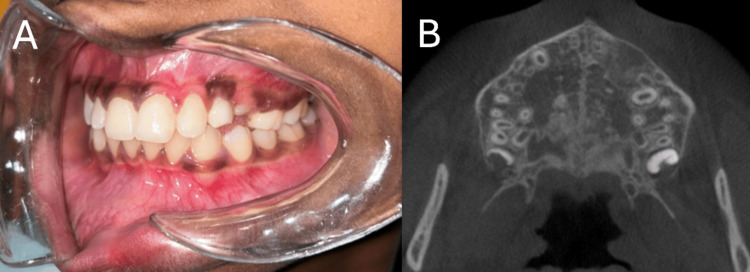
Postoperative images of six-month follow-up (A) Reduced vestibular obliteration and healed surgical site. (B) Adequate bone formation in the postoperative CBCT. CBCT: Cone beam computed tomography
